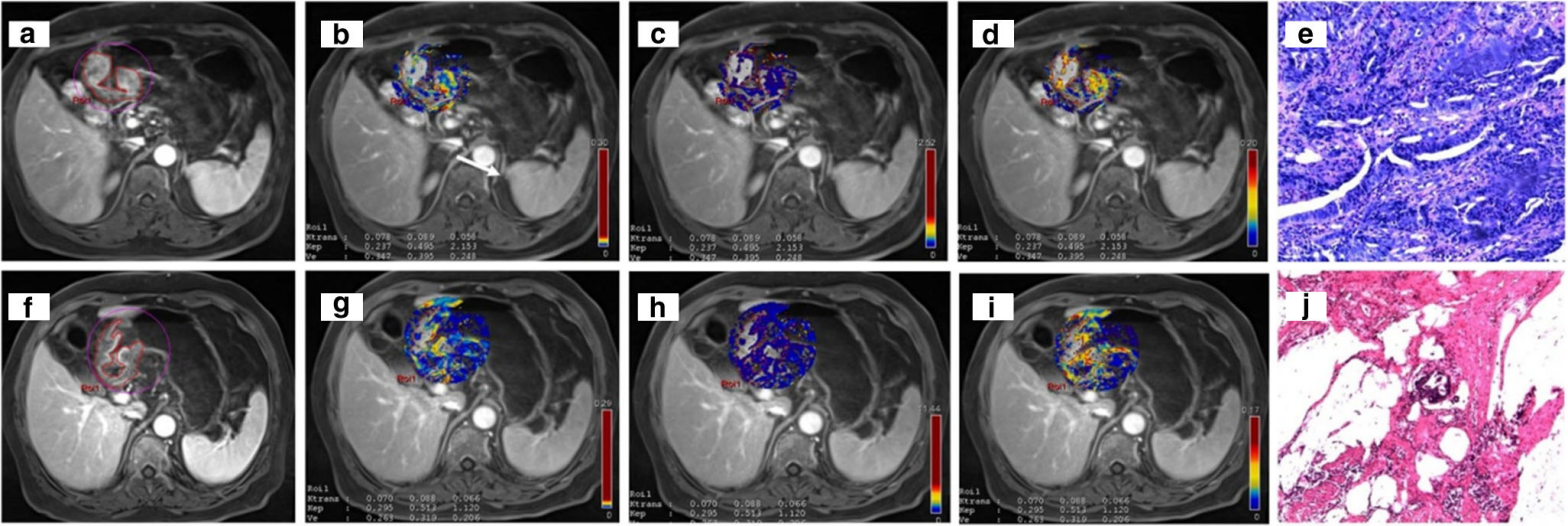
DCE-MRI showing a tumour of the gastric antrum (a) in a 66-year-old female. In the pretreatment scan, the K^trans^ (b) was 0.078 min^−1^, the K_ep_ (c) was 0.237 min^−1^ and the V_e_ (d) was 0.347. The tumour was confirmed at biopsy (e). In the posttreatment scan, there was a reduction in tumour size (f), and the K^trans^ (g) was 0.070 min^−1^, the K_ep_ (h) was 0.295 min^−1^ and the V_e_ (i) was 0.263. Final pathology (j): intestinal type (Lauren classification), staged as ypT1bN0 (tumour regression grade 1)
